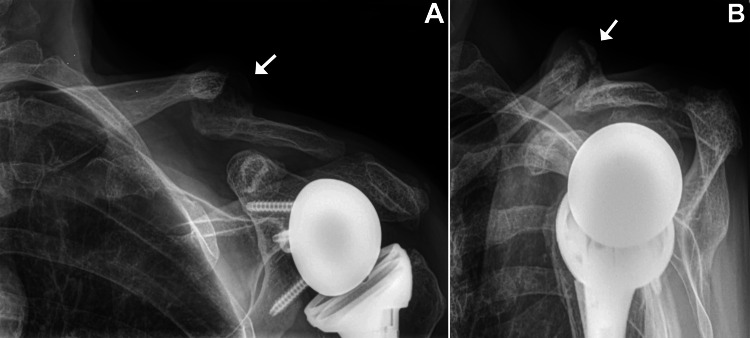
Radiographs taken 18 months after RTSA. Radiographs of the left shoulder taken 18 months after the RTSA (12 months after the occurrence of the clavicle fracture). (A) Anterior-posterior view and (B) scapula Y view. Arrows indicate the clavicle fracture non-union. RTSA: Reverse total shoulder arthroplasty
